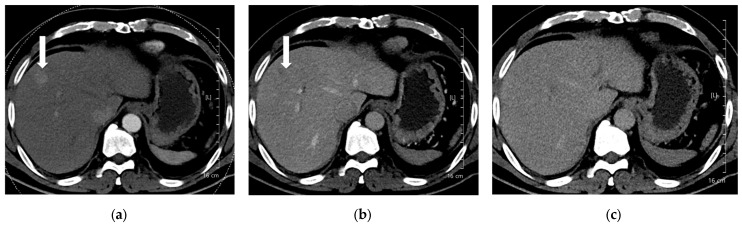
A 56-year-old man with hepatocellular carcinoma (HCC). CT was performed using low-concentration iodine contrast material (total iodine dose, 389.89 mg I/kg). Overall image quality in the arterial and portal phases was unacceptable (mean scores < 4): (a) A 17-mm enhancing nodule (arrow) is visible on the arterial phase at 120 kVp; (b) The nodule shows subtle washout on the portal phase (arrow); (c) The nodule is inconspicuous on the delayed phase. The two readers classified the lesion as LI-RADS 4 and LI-RADS 3, respectively.
